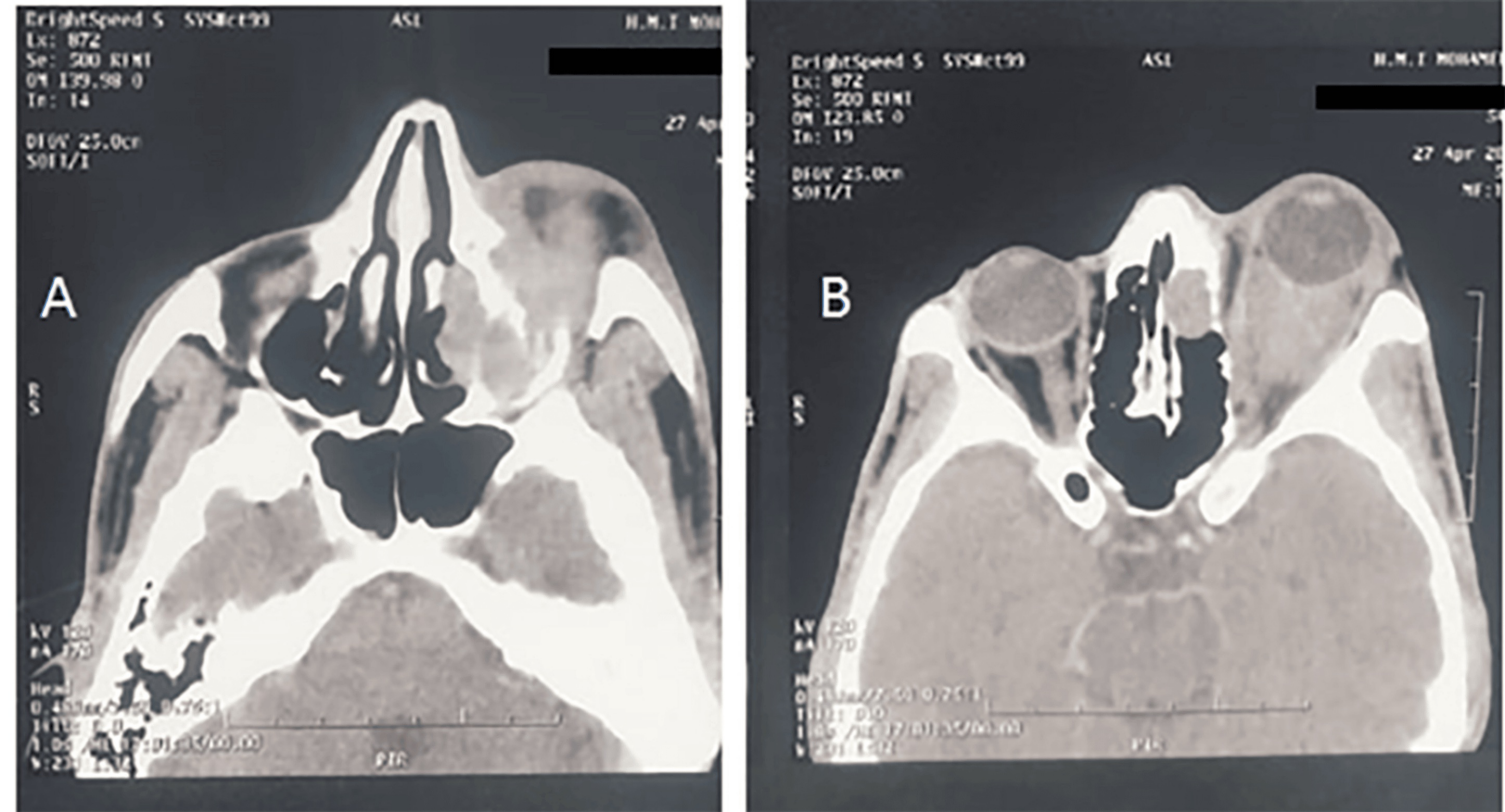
CT scan (axial slices) Presence of a roughly oval, well-defined, hypodense formation with thickened walls in the left orbit, measuring 20 x 16 mm, suggestive of an orbital abscess. This formation exerts a mass effect on the oculomotor muscles, causing grade 2 exophthalmos. Infiltration of the soft tissues and intra- and extraconal fat. This is associated with complete filling of the left maxillary sinus, frontal sinus, and lacrimal-nasal canal The image is suggestive of orbital cellulitis complicated by an orbital abscess, classified as Chandler stage IV
